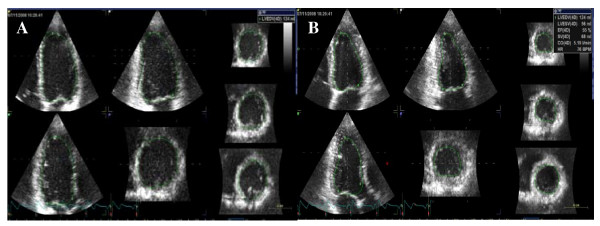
Three-dimensional echocardiographic ejection fraction measurement. Automate delineations in diastole (A) and systole (B) resulting in EF of 55%.
